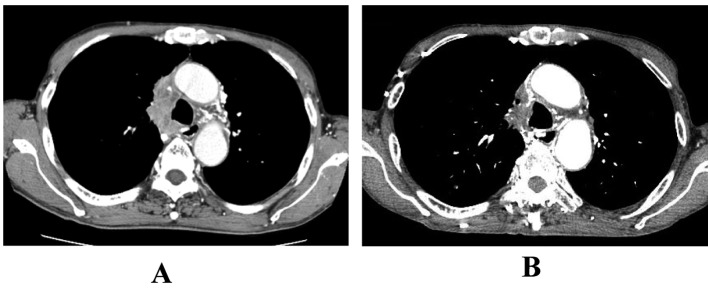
The response to second-line ADOC chemotherapy in case 2. Chest computed tomography before second-line ADOC chemotherapy (A) and after a cycle of second-line ADOC chemotherapy (B).
